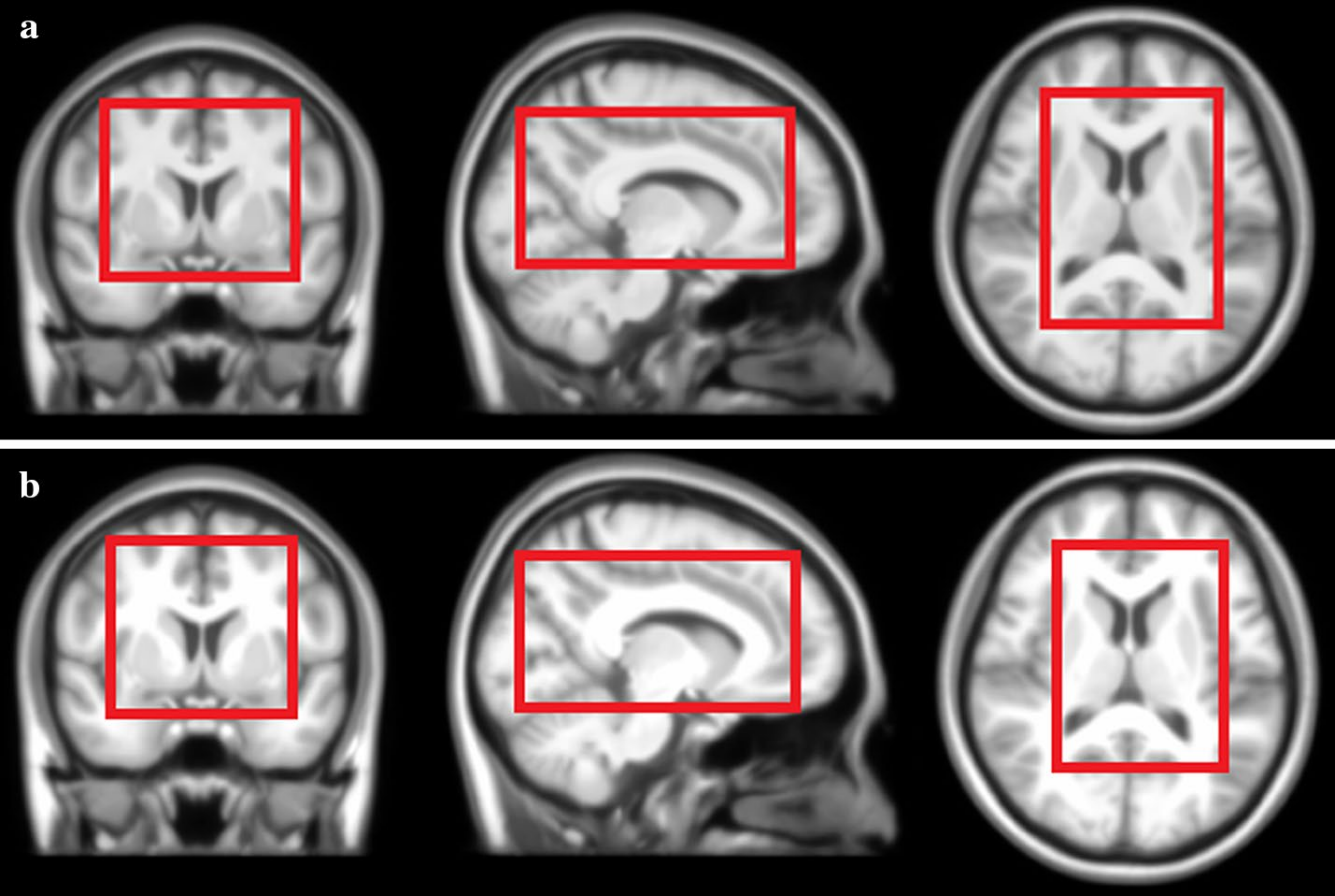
The average template of 100 Chinese adults (age ranged from 20 to 30 years) brain MR images from different scanners. a The average template without intensity normalization in the preprocessing procedure; b the average template after applying our histogram-based intensity normalization.
